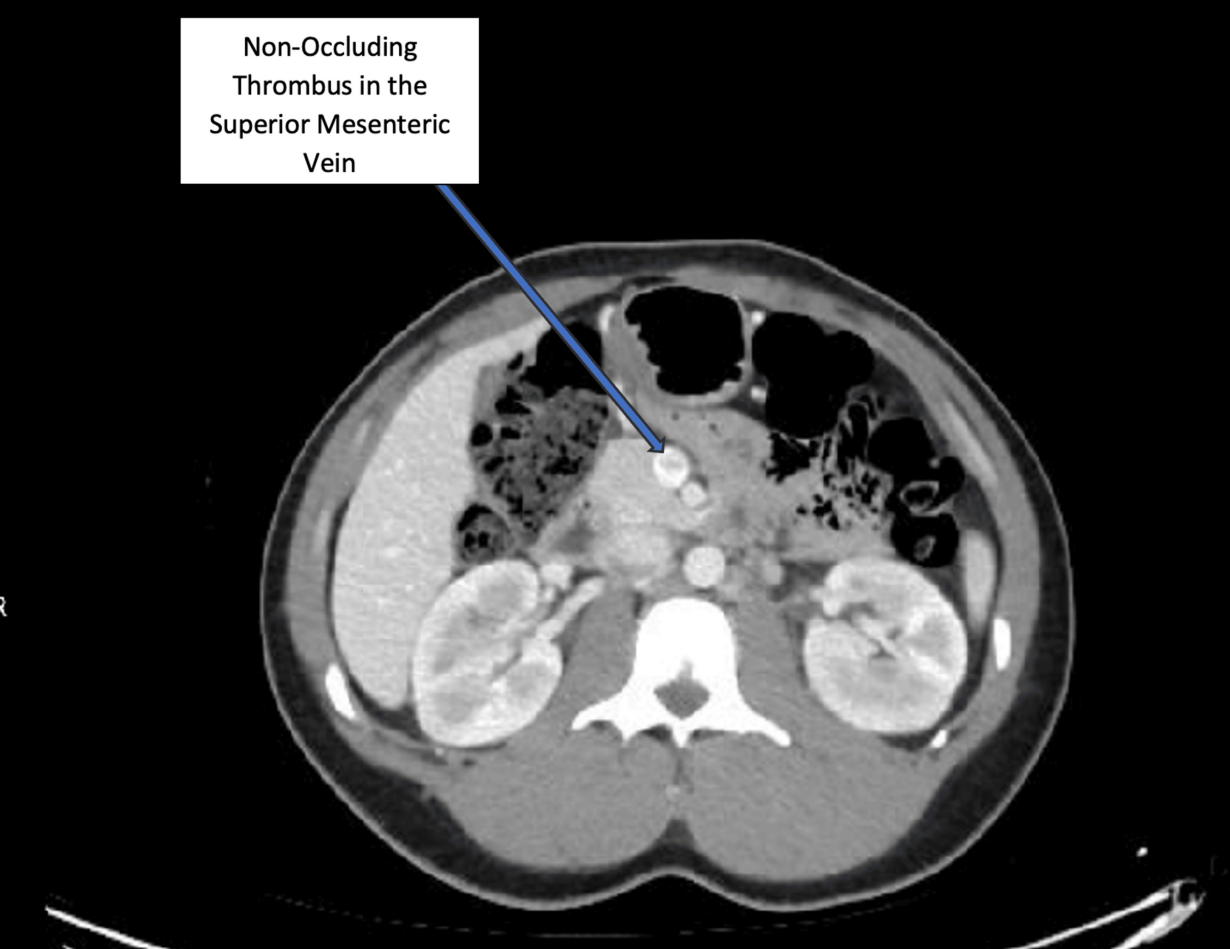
Computed Tomography of the abdomen with intravenous and oral contrast showing non-occluding thrombus in the superior mesenteric vein.
